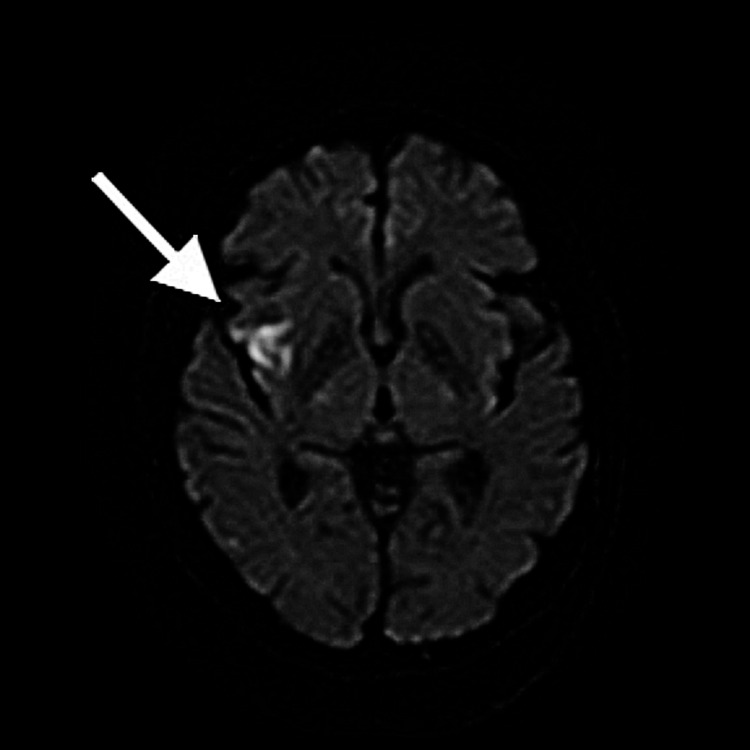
Brain MRI image Brain MRI on admission in Case 1 shows a new infarction in the upper right cortex
